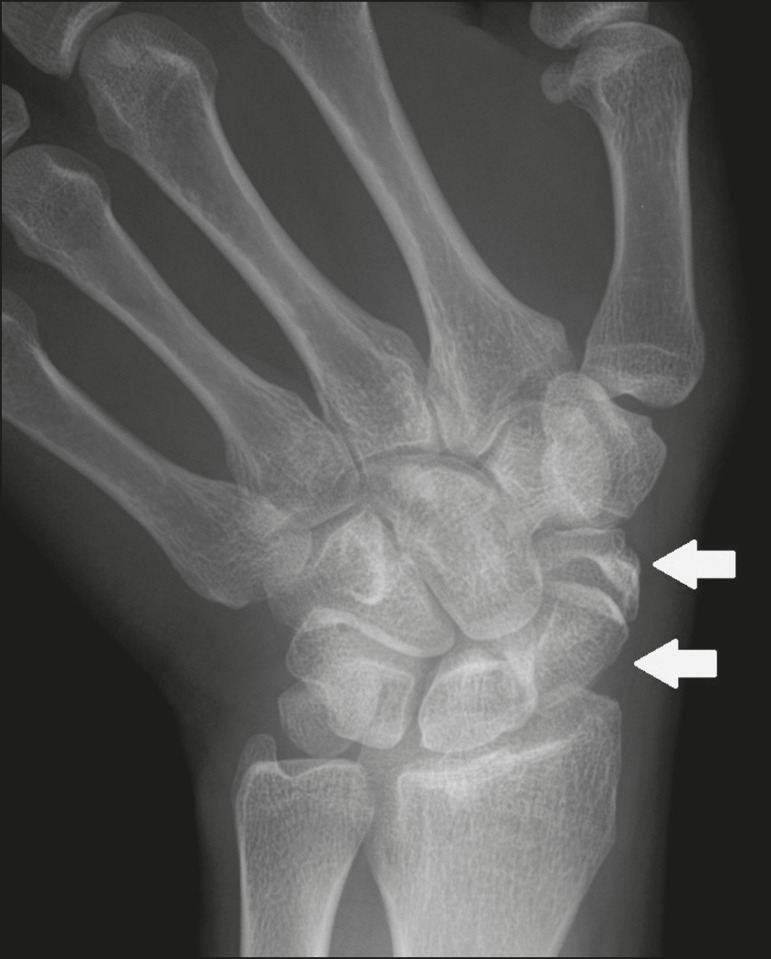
X-ray of the right wrist in ulnar deviation showing a divided scaphoid
